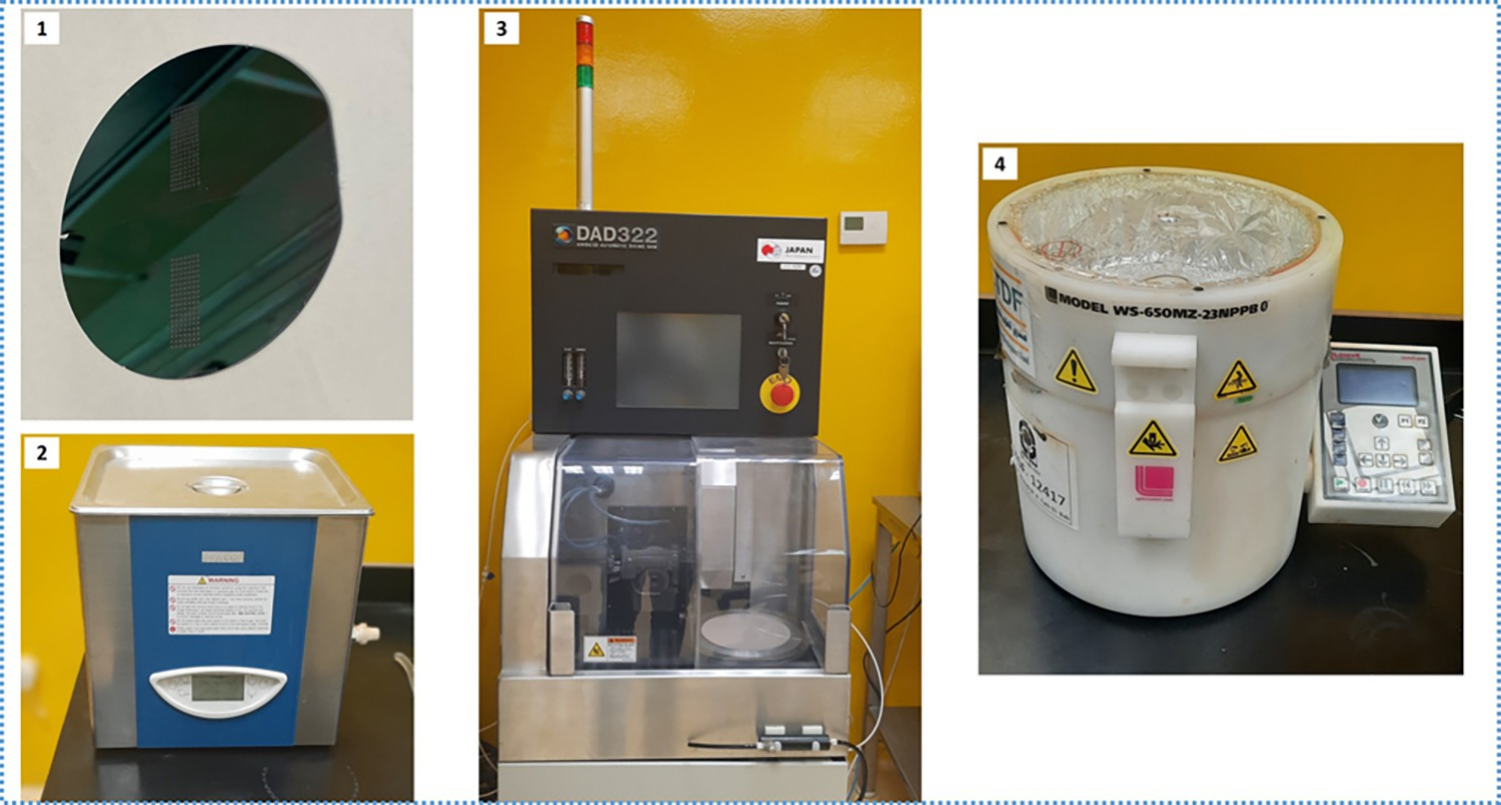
Substrate preparation: (1) Silicon wafer, (2) Ultrasonic cleaner (LABSONIC LBS2-4,5), (3) Dicing saw (DAD322) and (4) Spin coater (WS-650-23B).
